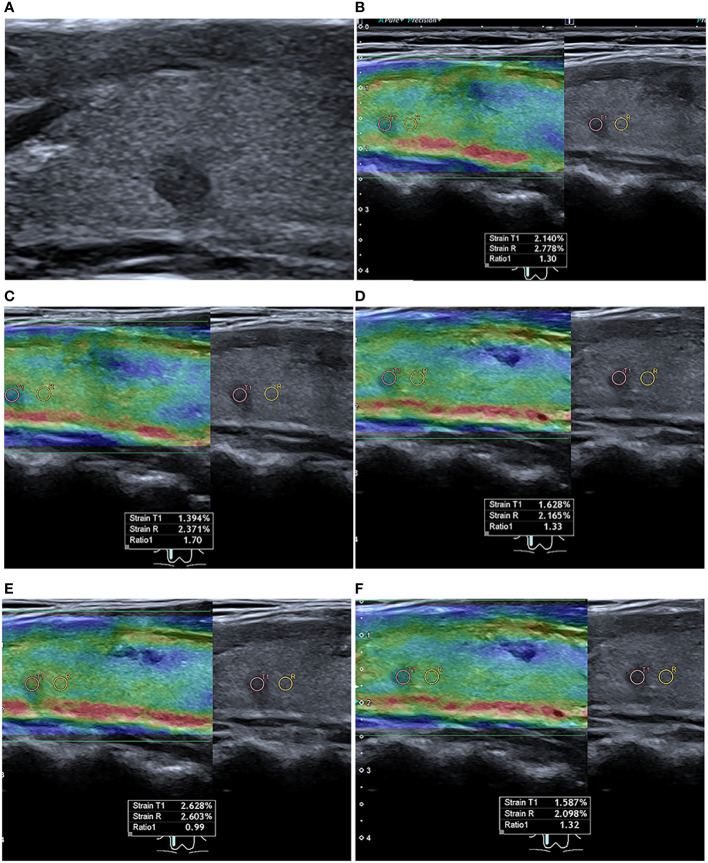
Conventional and strain ultrasound elastography of a 45-year-old female PTC patient without occult central lymph node metastasis. (A) Conventional ultrasound shows a hypoechoic nodule in the upper right lobe of the thyroid gland with a maximum diameter of 4.70 mm. (B–F) Five strain ultrasound elastography images were obtained, with strain ratios of 1.30, 1.70, 1.33, 0.99, and 1.32, respectively.
